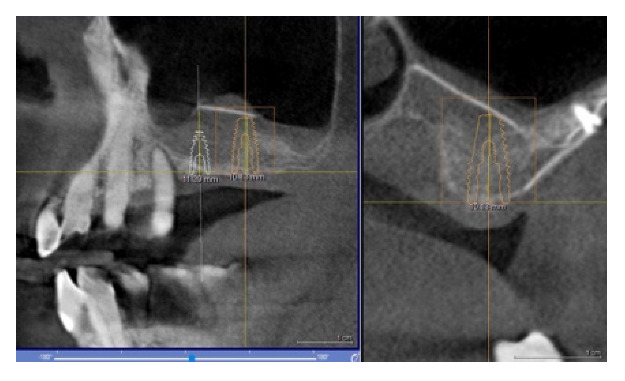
Foil radiographic checks: in evidence the shape of the foil.
